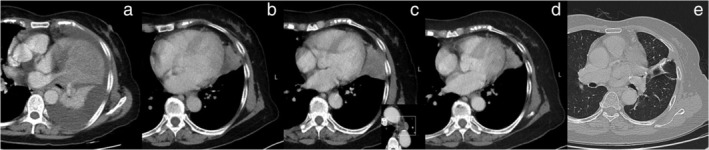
Chest computed tomography (CT) of the patient. (a) Chest CT showed an ill‐defined left lung mass with obstructive atelectasis and massive pleural effusion. (b) Follow‐up CT after platinum‐based doublet chemotherapy (cisplatin plus gemcitabine) for six cycles revealed a notable shrinkage in the left lung tumor (maximum tumor diameter 4 cm). (c) Follow‐up CT revealed evidence of progressive disease for the enlargement of mediastinal lymphadenopathies and the primary tumor (maximum tumor diameter 5.5 cm). (d) Follow‐up CT (just before the surgery) after immunotherapy showed partial response (maximum tumor diameter 3.0 cm). (e) Follow‐up CT 16 months after salvage surgery revealed no evidence of recurrence
